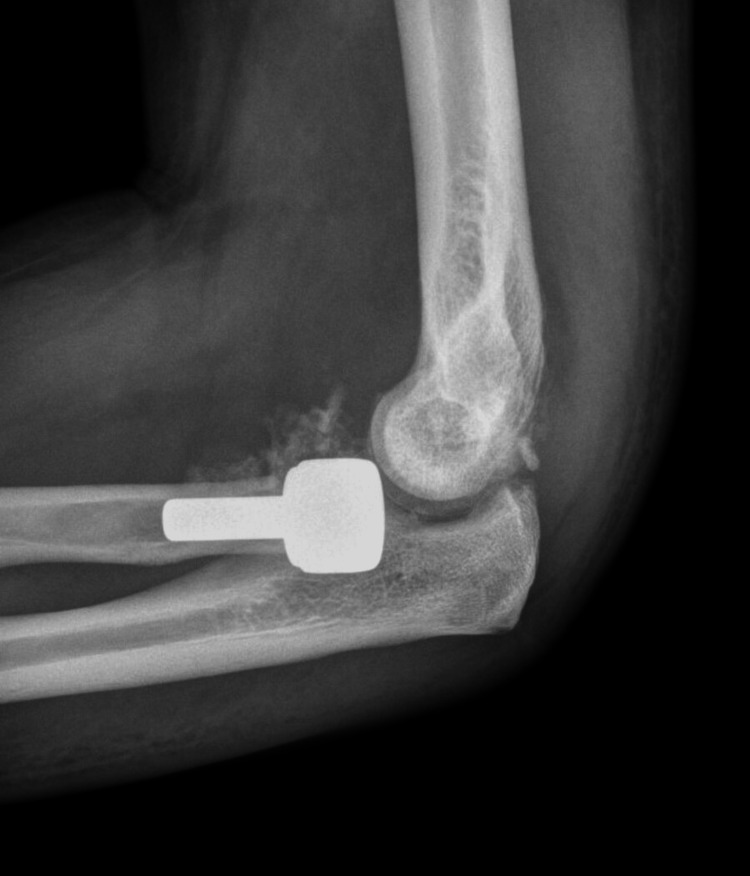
Lateral elbow radiograph demonstrating posterior subluxation of the radial head prosthesis 4 weeks after the index TT procedure. TT, terrible triad
